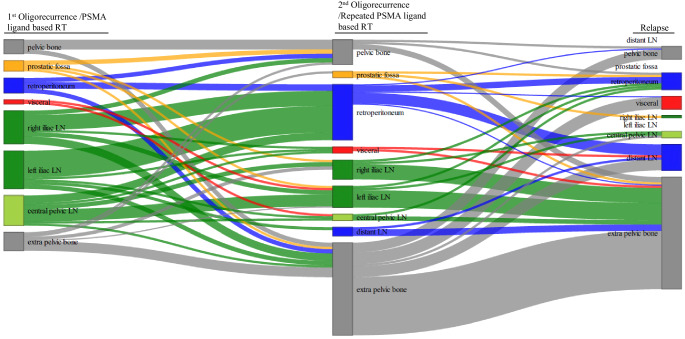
Sankey plot showing the location of new metastases in relation to prior metastatic sites (for every patient, every metastatic lesion was counted). On the left side, the initial distribution at oligorecurrence at the time of first PSMA PET-directed RT is shown; in the middle, the distribution of metastases at the time of second PSMA PET-directed RT is shown; and on the right side, the distribution after second PSMA ligand-directed RT is shown. Similar to the analysis of the involved anatomic regions (Fig. 2), we observed a numerical shift from iliac lymph node metastases to retroperitoneal lymph node metastases and marked skeletal involvement, particularly involving the extrapelvic skeletal system. *PSMA* prostate-specific membrane antigen, *RT* radiotherapy, *LN* lymph node
